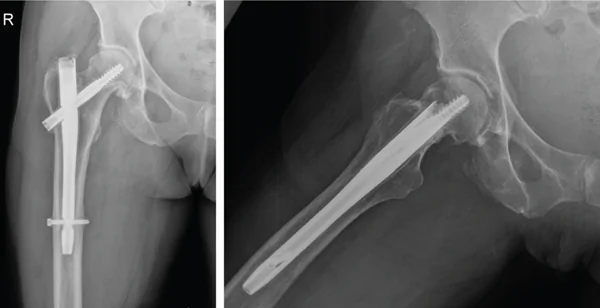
AP and lateral radiographs at 6 months following subsequent injury.
